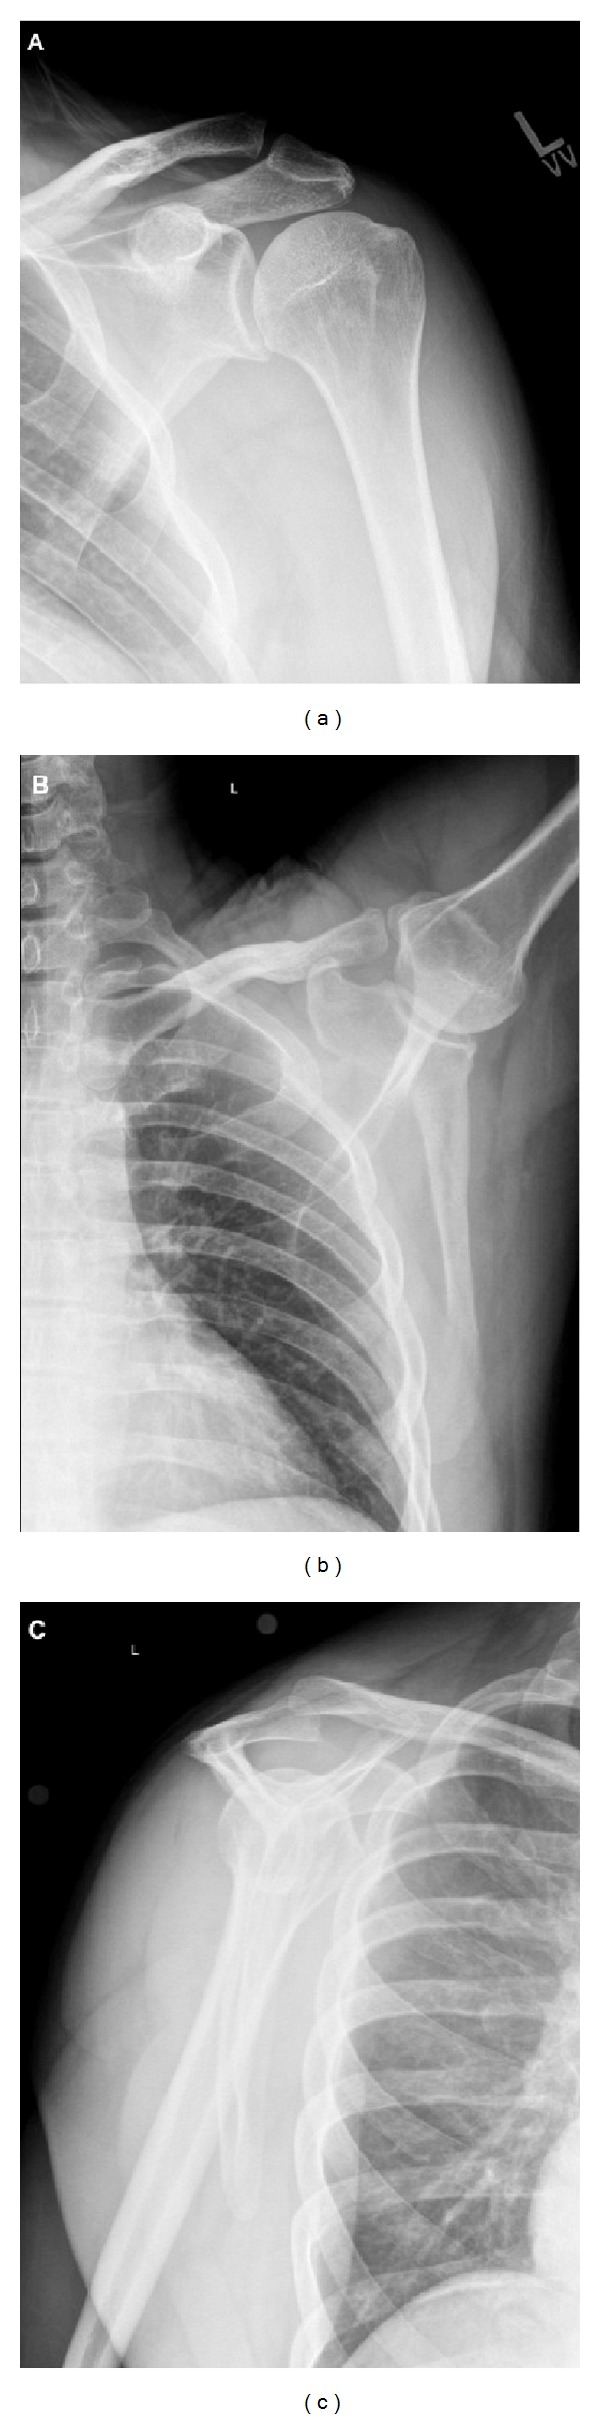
Radiographs including (a) AP, (b) axillary, and (c) scapular Y of the left shoulder.
